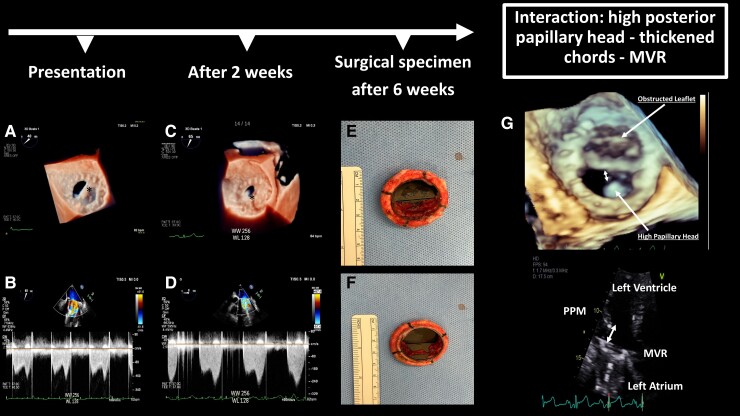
A transoesophageal echocardiogram (TOE) revealed a non-mobile mass adjacent to the posteromedial occluder causing restricted opening and partial obstruction of the MVR (*Panels A* and *B*; *Videos 1* and *2*). Blood cultures were negative including for fastidious microorganisms. Treatment with unfractionated heparin and antibiotics was given empirically for 2 weeks, and although inflammatory markers normalized, repeat TOE showed a progression to severe MVR obstruction with increased mass size now partially restricting also the anterolateral occluder (*Panels C* and *D*; *Videos 3* and *4*). Persistent obstruction on transthoracic imaging was subsequently demonstrated and re-sternotomy surgery was therefore advised. Surgical inspection revealed a laminated thrombus on the posteromedial occluder of the MV prosthesis (*Panels E* and *F*).
